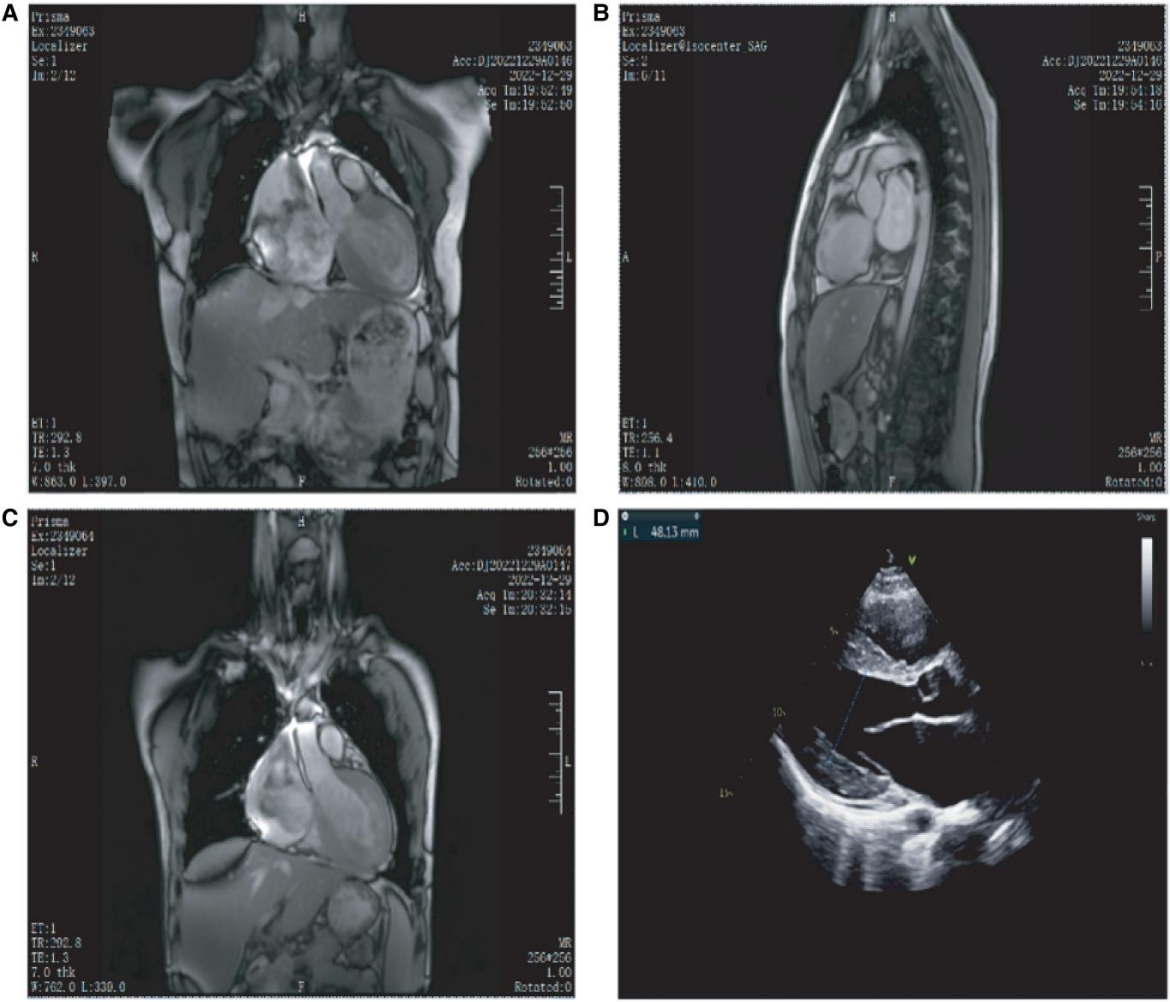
Imaging findings in the proband and his brother. (*A* and *B*) Cardiac MRI findings in the proband. (I) Cardiac MRI findings in the proband’s brother. (*D*) Cardiac ultrasound findings in the proband.
